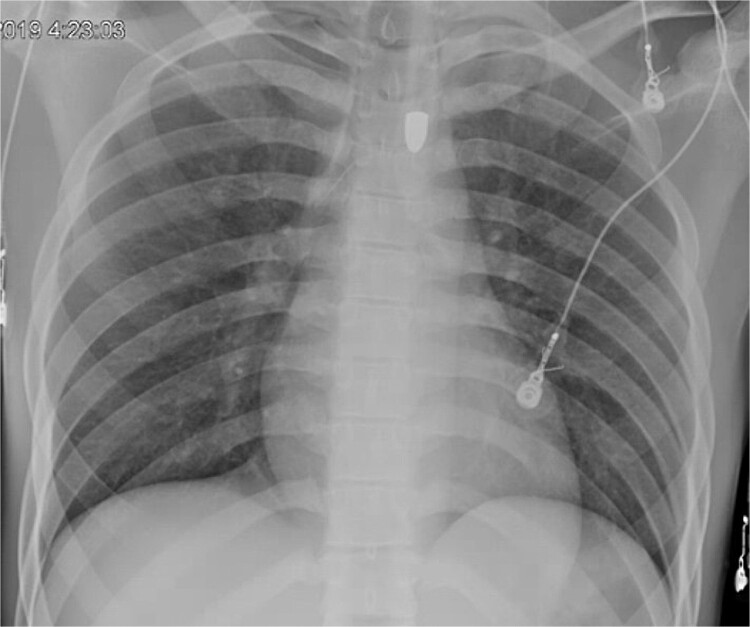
Trauma bay chest X-ray showing retained projectile.
